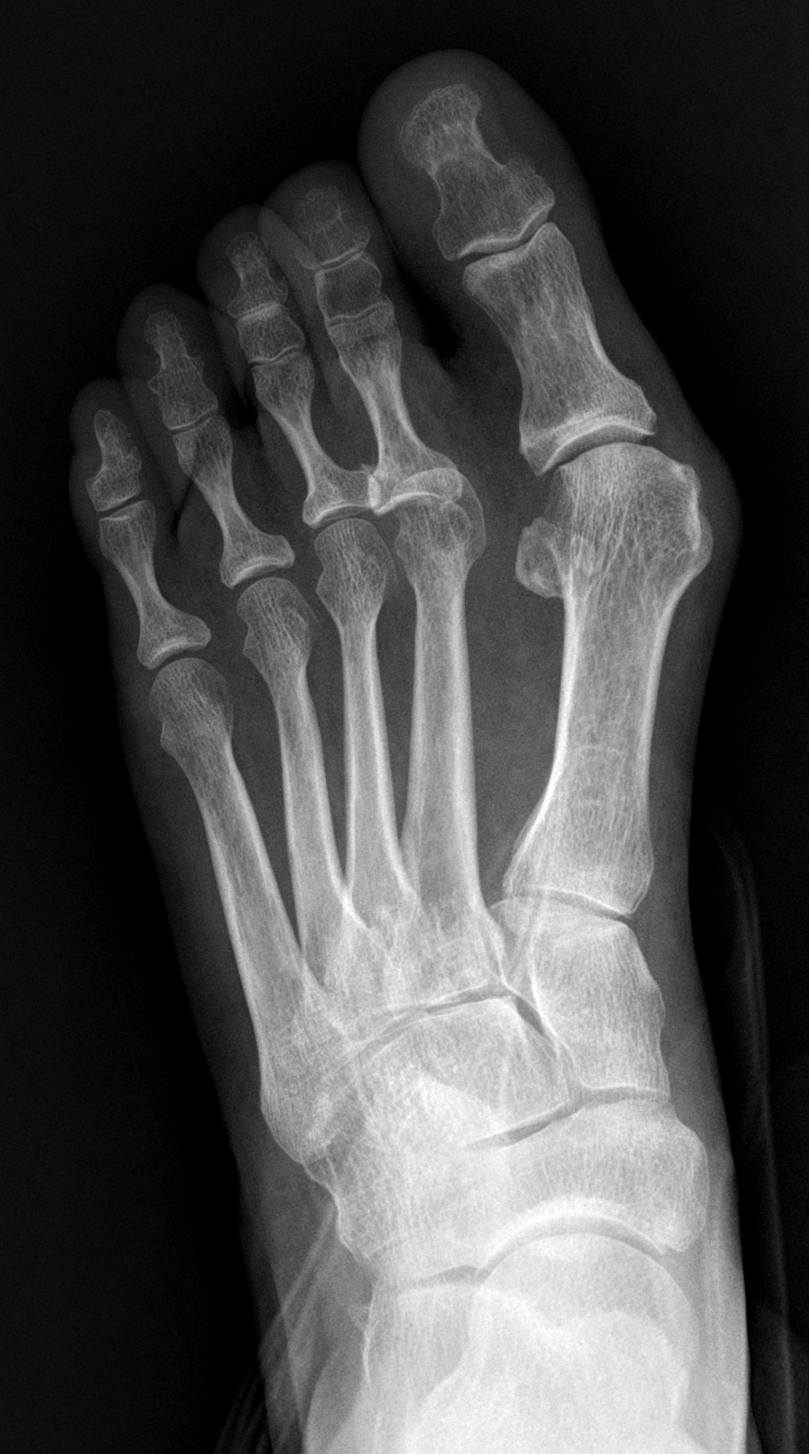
Preoperative weightbearing X-ray of a 55-year-old female. Notice the excessive length of the great toe and protrusion of the first metatarsal.
